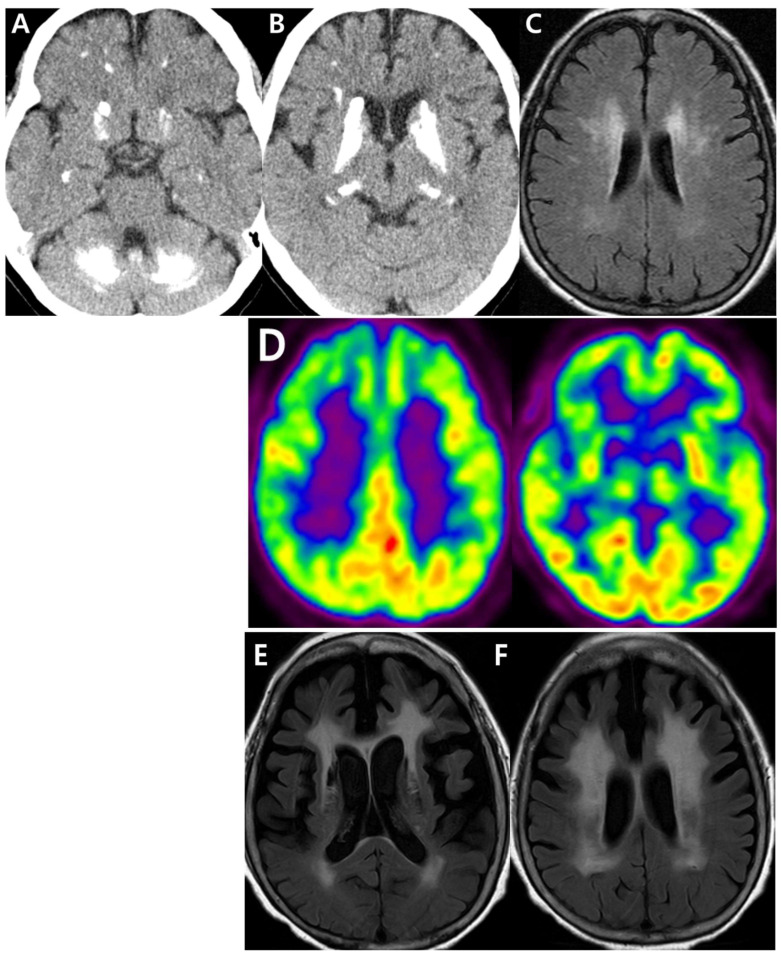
Imaging data of proband patient. (A,B) CT imaging of the patient at the age of 61 revealed calcification in the bilateral subcortical white matter, basal ganglia, thalami, and dentate nuclei. (C) FLAIR-MRI imaging at her initial visit showed severe diffuse periventricular white matter hyperintensities. (D) PET imaging showed hypometabolism in the frontal, temporal, parietal cortex, basal ganglia, and right thalamus. Red meant the high brain metabolism, green meant the moderate brain metabolism, while blue and purple meant the reduced brain metabolism (E,F). MRI FLAIR images obtained at the age of 77, with observed progression of periventricular white matter hyperintensities.
